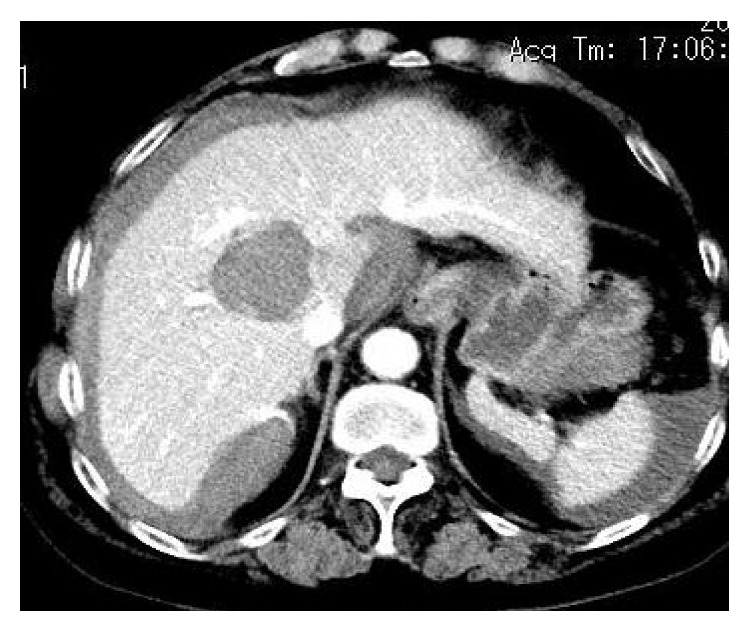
Abdominal CT revealed rupture of the posterior lobe lesion, hemorrhage around the liver, and accumulation of fluid in the abdominal cavity and pelvic cavity.
